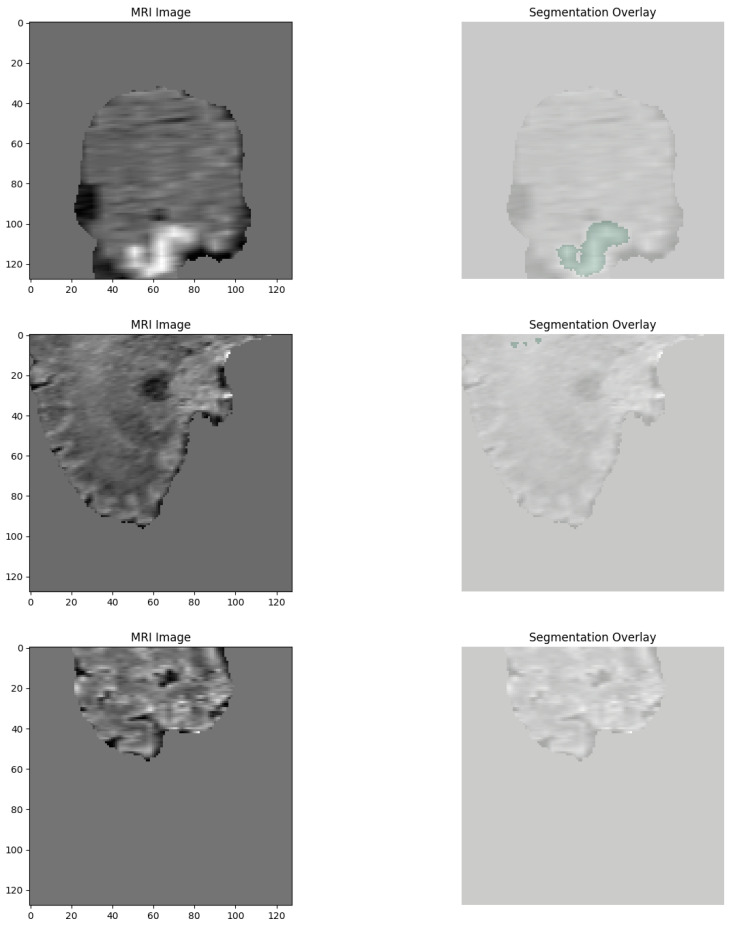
Representative 3D MRI samples with segmentation overlays for TC, WT, and ET.
